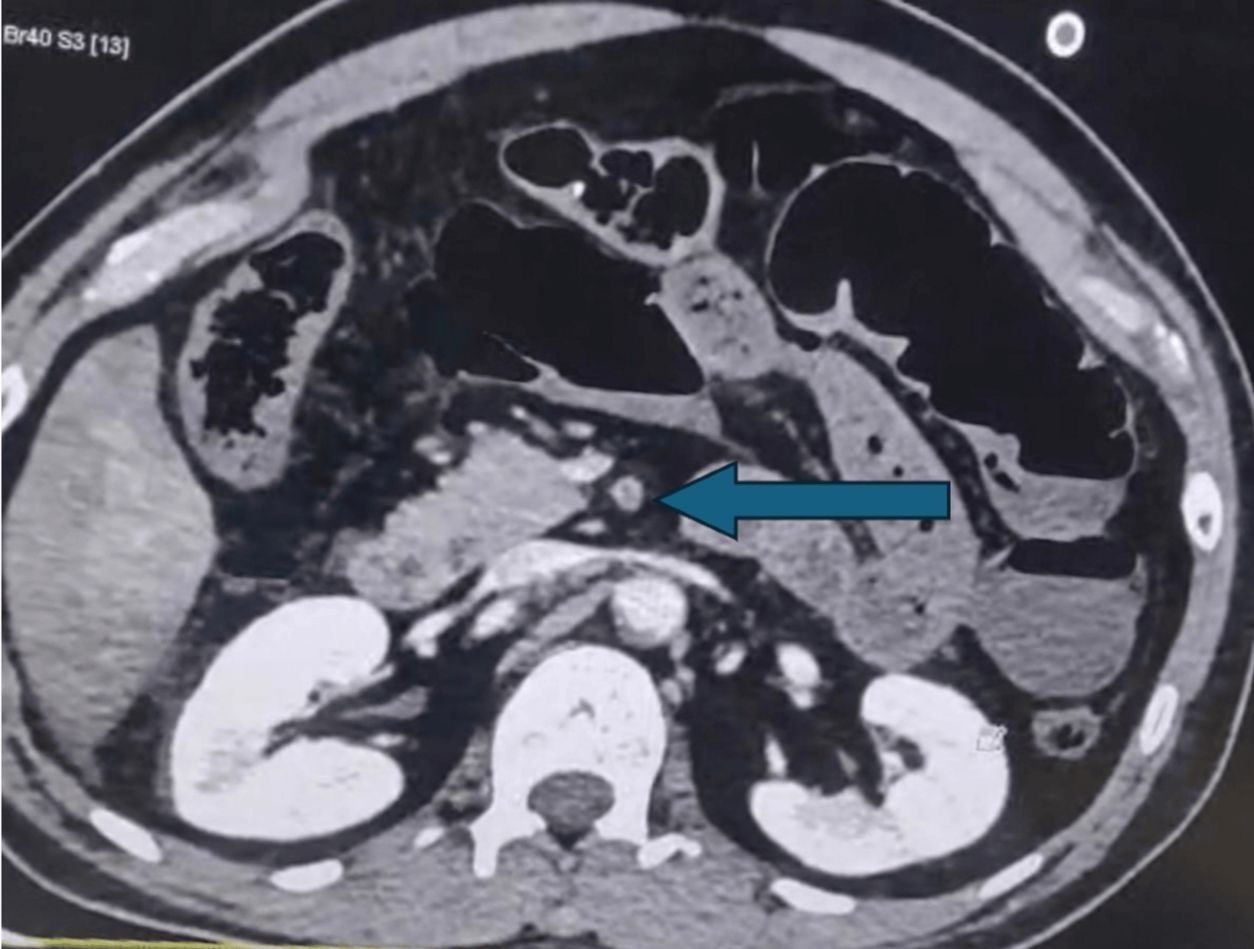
Axial contrast-enhanced computed tomography (CECT) image revealing proximal superior mesenteric artery (SMA) thrombosis The blue arrow points to a hypodense luminal filling defect within the proximal superior mesenteric artery, indicating acute thrombotic occlusion. Adjacent vascular segments appear narrowed, supporting the diagnosis of diffuse mesenteric ischemia.
